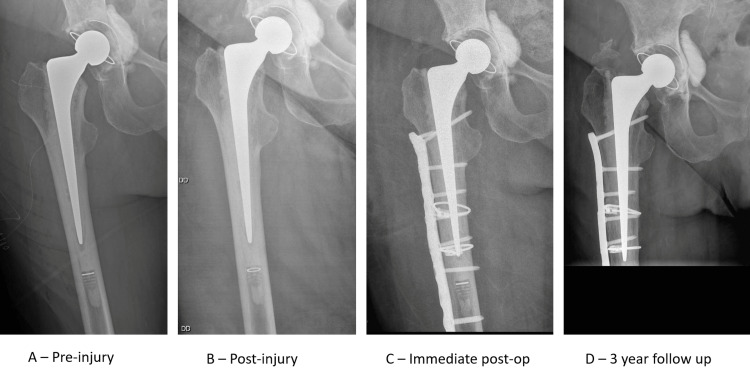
50% rule and further subsidence (A) Pre-injury AP radiograph; (B) AP radiograph taken post-injury demonstrating positive ‘SINC’ sign with >50% obliteration of lucency representing the centraliser when compared with pre-injury radiograph; (C) immediate post-operative radiograph demonstrating stem position following plate osteosynthesis; (D) AP radiograph at three year follow up demonstrating significant further stem subsidence.
